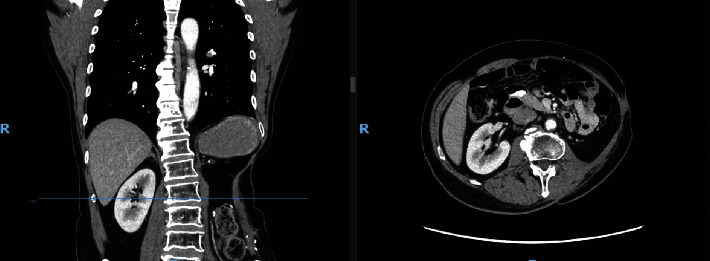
Postoperative CT scan of patient at 2-year mark. Note: CT scan of the lateral abdominal wall reconstruction at 2 years postoperatively.
